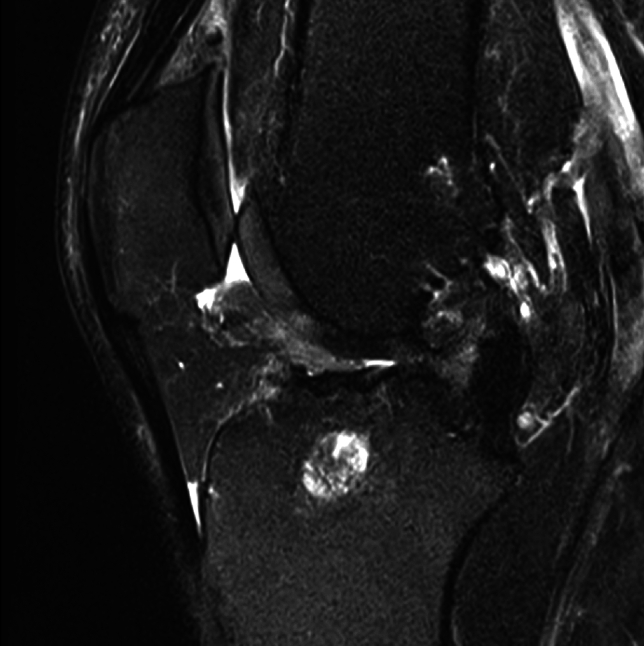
42-year-old female patient at follow-up MRI 7 months after QT autograft ACLR showing complete graft resorption and missing graft signal 7
